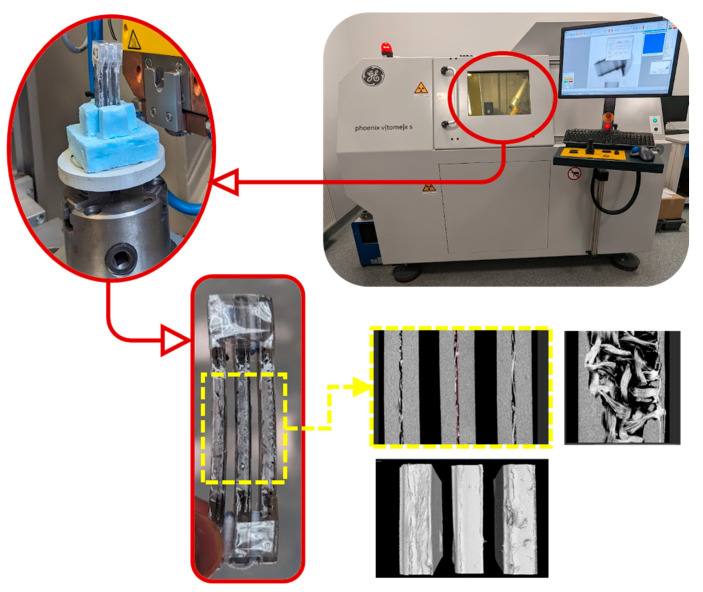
Computed Tomography scan of a set of three samples.
